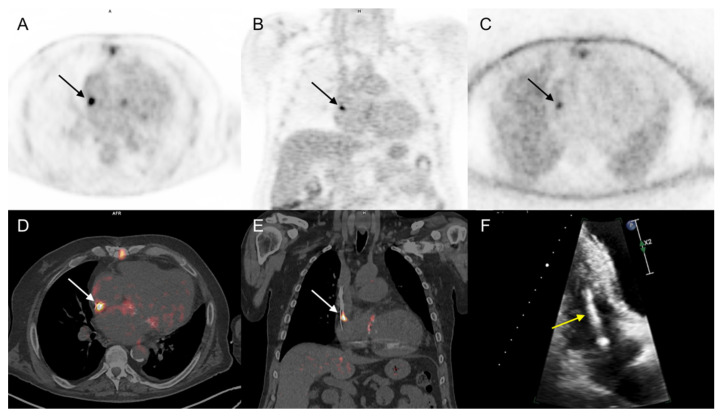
68-year-old man with a history of implantable cardioverter defibrillator (ICD) and a biological prosthetic mitral valve presented with 10-mm enlargement of ICD lead within the right auricle at TTE (yellow arrow) but normal routine biological evaluation and negative blood cultures (Duke 1/high clinical suspicion degree). PET/CT showed increased focal ^18^F-FDG uptake, corresponding to right auricle ICD lead (arrows). The Endocarditis Team’s final diagnosis was CIED IE, and ICD was removed. (A,B): PET, attenuation-corrected axial, and coronal slices. (C): PET, non-attenuation corrected axial slice. (D,E): PET/CT, axial, and coronal attenuation corrected slices. (F): TEE.
